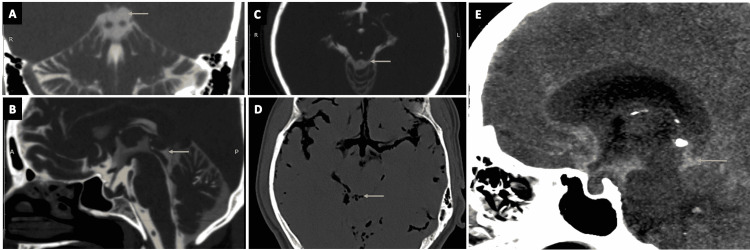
The cisterna quadrigeminalis (quadrigeminal cistern) (continued). (A), (B), and (C) show the quadrigeminal cistern (arrows) as visualized in the coronal, sagittal, and axial sections of CT cisternography, respectively, in a case of suspected CSF rhinorrhoea. (D) The quadrigeminal cistern (arrow) as seen in an axial plane of CT brain (negative contrast) in a case of pneumocephalus. (E) The quadrigeminal cistern (arrow) as seen on the sagittal plane of CT brain (positive contrast) in a case of subarachnoid hemorrhage (SAH).
